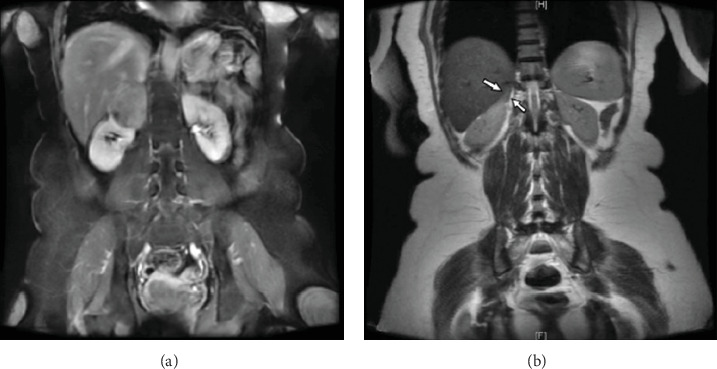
(a) The renal parenchyma can be seen creeping alongside the mass, a finding known as the “claw” sign, and is consistent with a mass of renal origin. (b) The right adrenal gland was identified on MRI and noted to be distinct from the renal mass.
